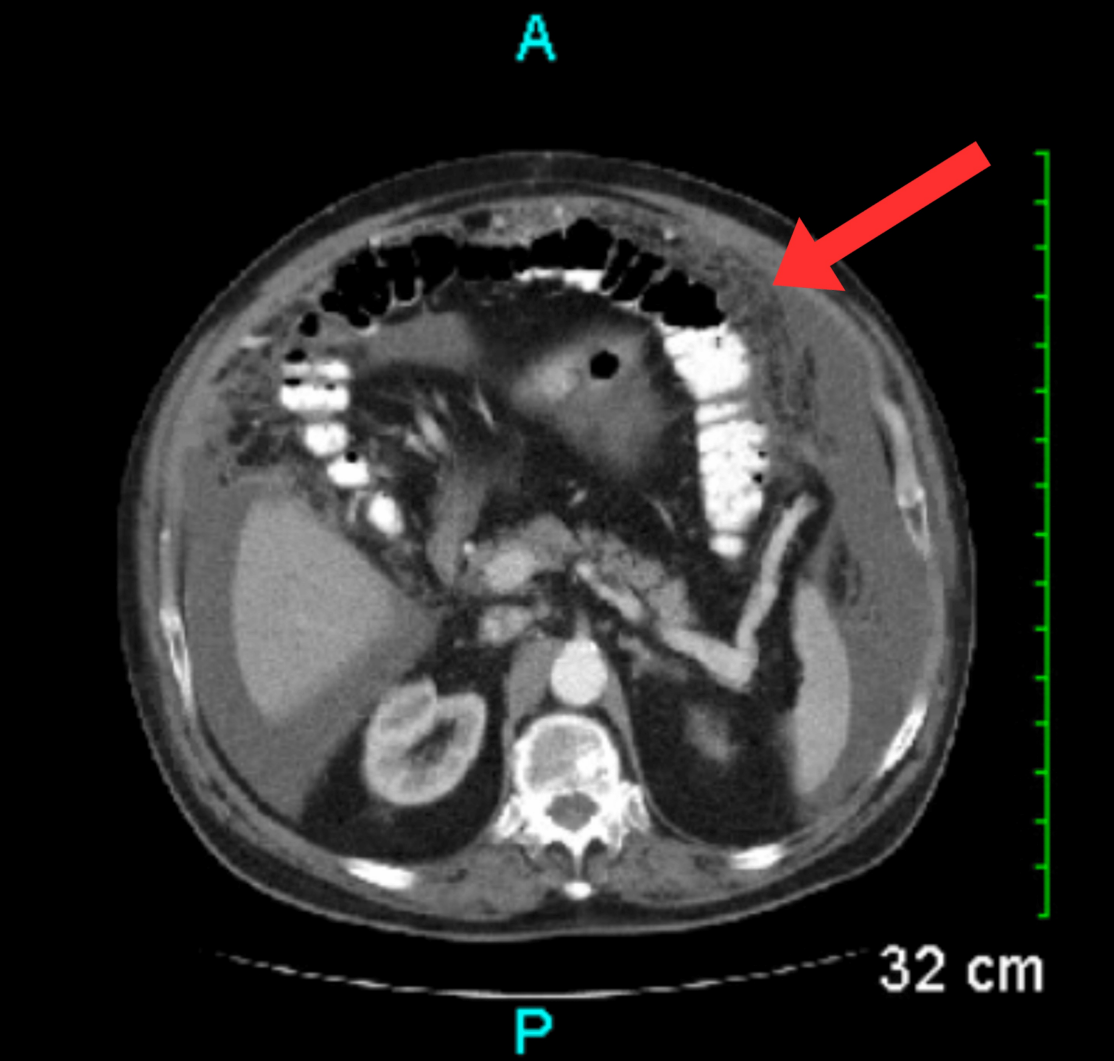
CT scan showing moderate diffuse omental edema (red arrow). CT: Computed tomography
